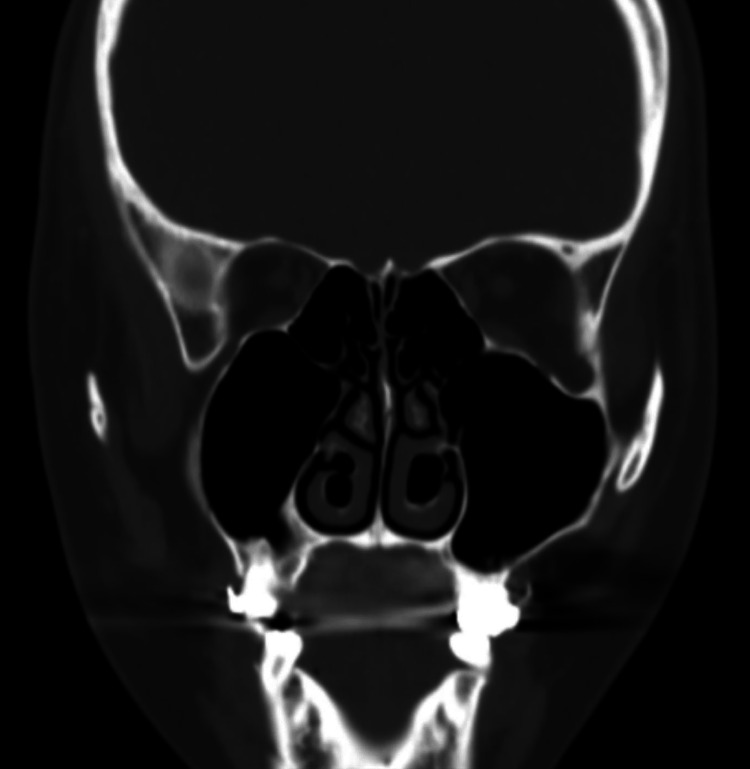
Non-contrasted paranasal sinuses CT scan, coronal view for case 3. It shows bilateral inferior turbinate pneumatization of the bulbous type with communication to the inferior meatus.
